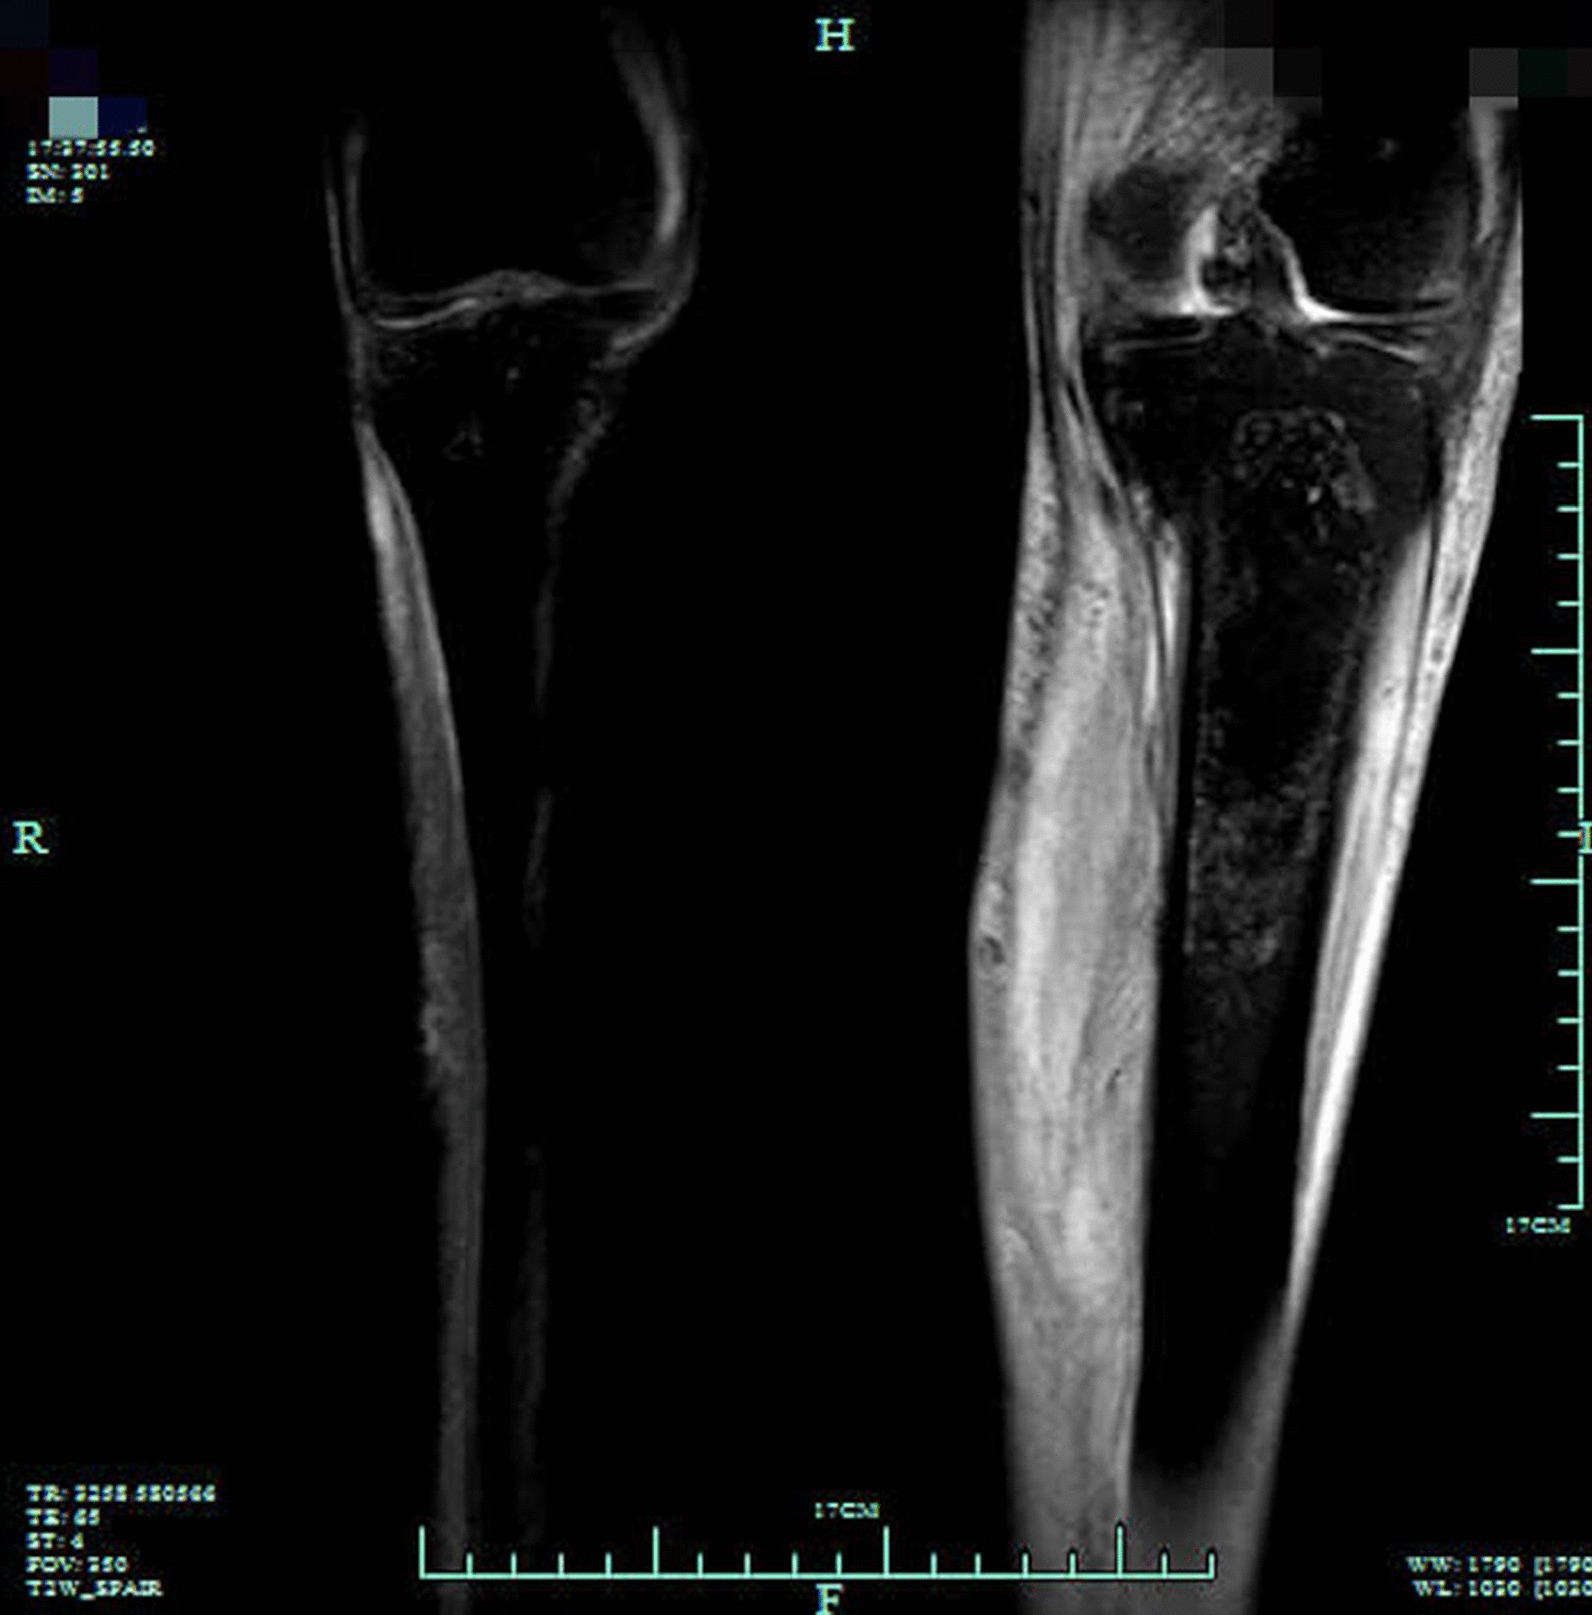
Bilateral knee joint MRI examinations show joint effusion in the left knee, with enhanced T1 signals in the medullary cavity above the tibia, consistent with bone infarct
